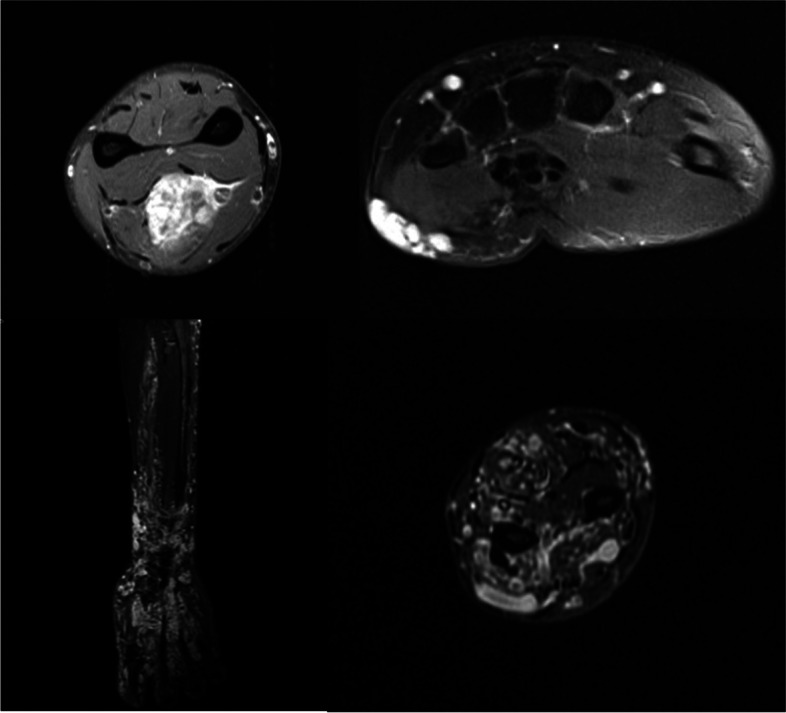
Aspect of a well-defined (top) and diffuse (bottom) VM on MRI
